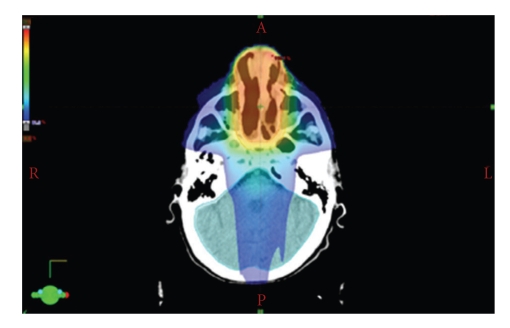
Radiotherapy planning I*. IMRT plan with the planning target volume (PTV) in one CT cross section, red colours indicate a higher dose; a steep dose gradient can be achieved by using fluence modulation with a multi leaf collimator. Normal tissues are optimally spared.
